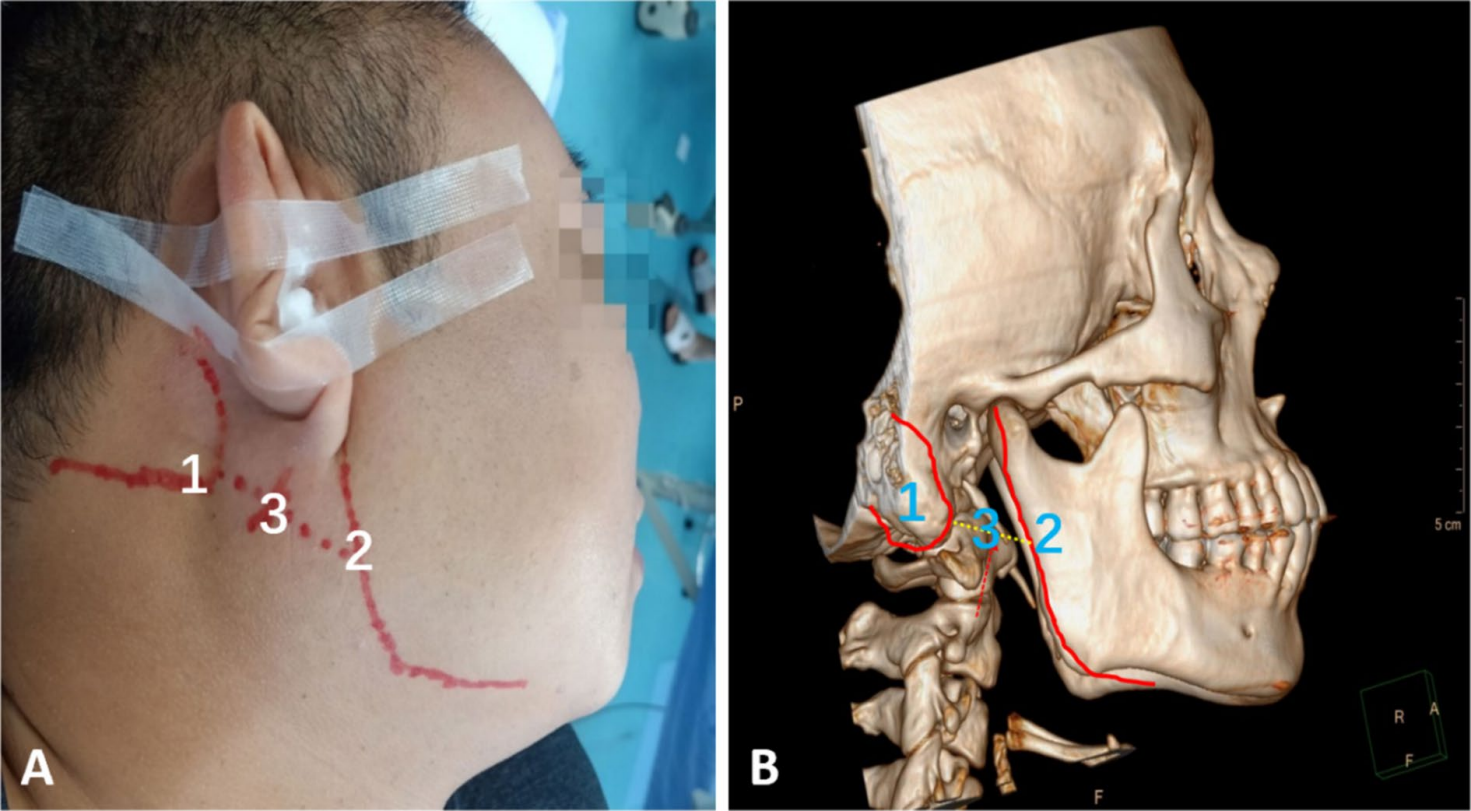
Patient’s body position and body surface markers (A) Body positioning: patient in a supine position with the head tilted to the opposite side. Label 1 indicates the mastoid, label 2 indicates the mandibular margin, and label 3 indicates the midpoint between the mastoid and mandibular margin. (B) CT 3-dimensional reconstruction of panel (A).
